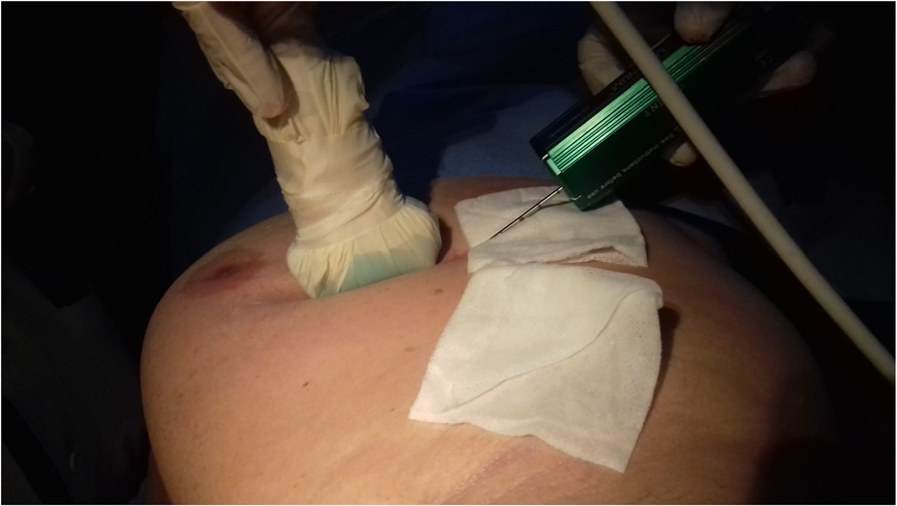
Ultrasound-guided core needle biopsy. The patient is in supine position. After local anaesthesia, the ultrasound probe (on the left) guides the needle to the lesion
